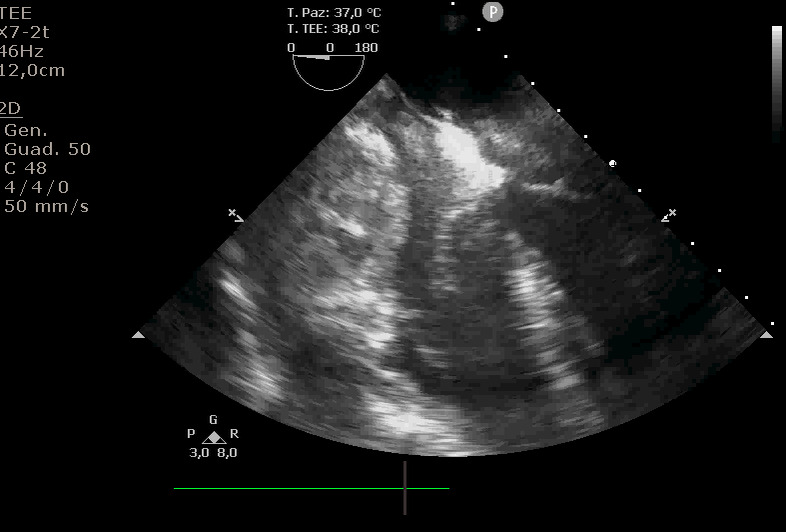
Transesophageal echocardiography (mid-esophageal four-chamber view) showing pericardial effusion with intrapericardial clot causing right-sided chamber compression. Mid-esophageal four-chamber TEE image demonstrating pericardial fluid with echogenic organized material consistent with clot, associated with focal external compression of the right atrium and right ventricle—an imaging pattern typical of regional/loculated postoperative tamponade and one that may be underestimated on transthoracic windows.
